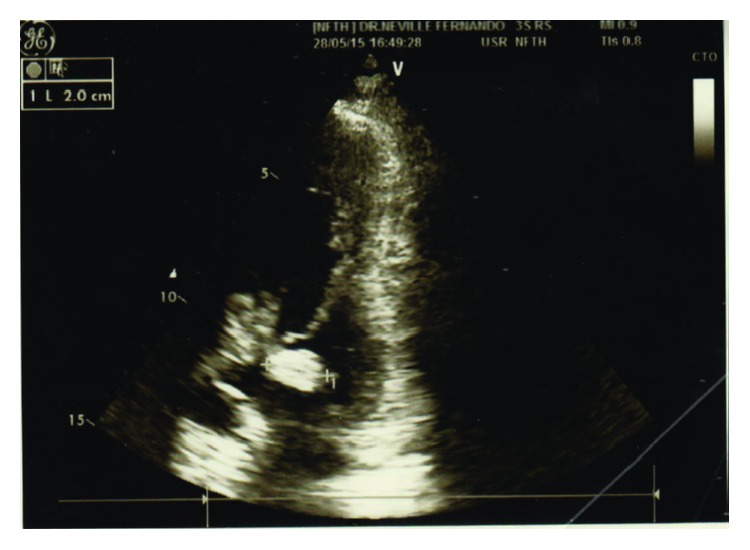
2D echocardiogram showing large sessile vegetation attached to anterior mitral valve leaflet.
